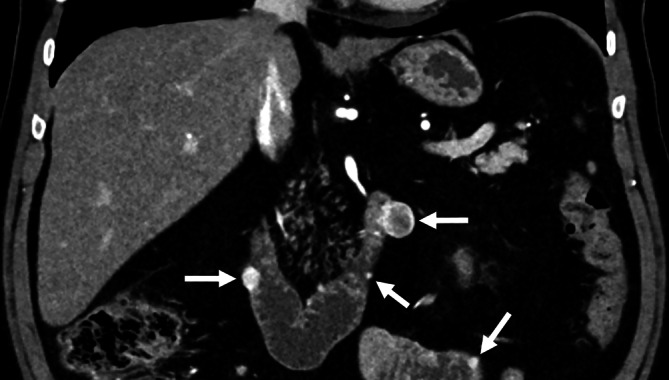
53-jähriger Patient mit Neurofibromatose Typ I ohne abdominale Symptome. Die Computertomographie (CT) in der arteriellen Phase zeigt mehrere hypervaskularisierte, scharf begrenzte, exophytische Läsionen von Duodenum und Jejunum (*Pfeile*), assoziierten GIST(gastrointestinale Stromatumoren)-Tumoren entsprechend
